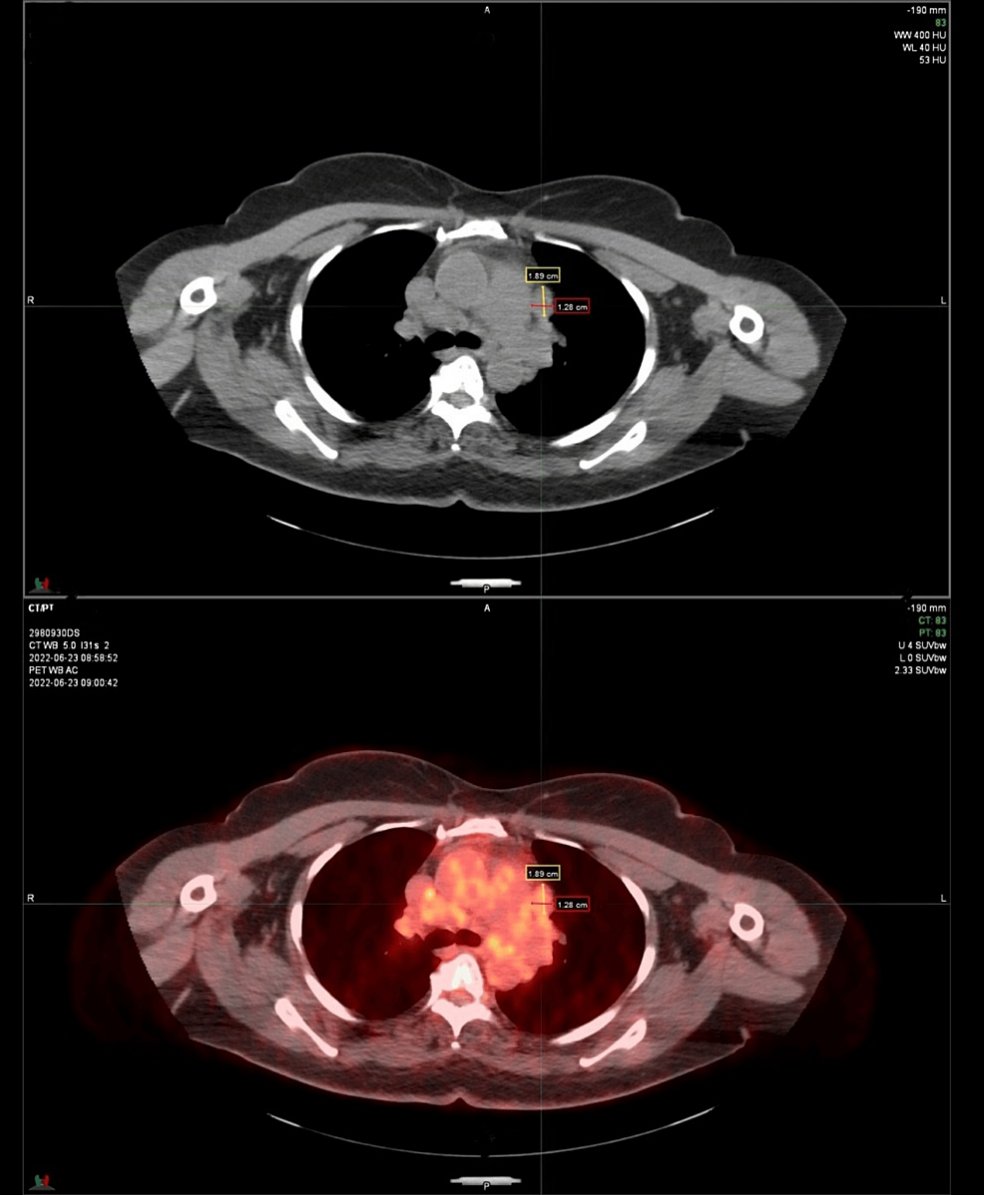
The PET-CT scan of the chest revealed a 2.8 cm mildly hypermetabolic nodular opacity in the left upper lung lobe and a 0.6 cm nodular opacity near the right major fissure with mildly hypermetabolic mediastinal and bilateral hilar lymph nodes and no enlarged lymphadenopathy in the neck, chest, abdomen, and pelvic areas.
